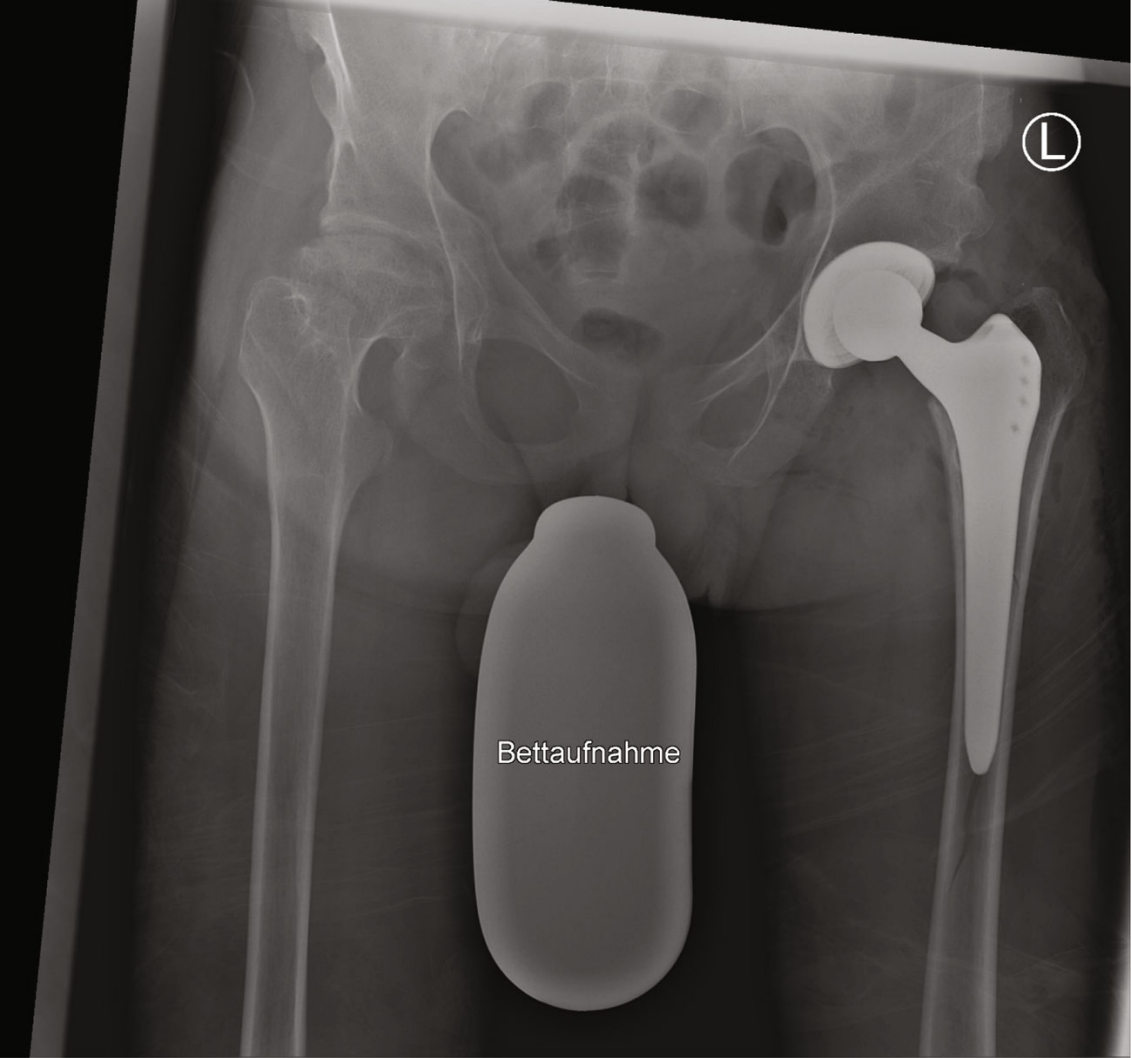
Postoperative image with periprosthetic fracture. This X-ray was taken in the postoperative care unit while the patient was lying in bed. A Vancouver type B1 fracture can be detected on the image. Hence, revision surgery was planned.
